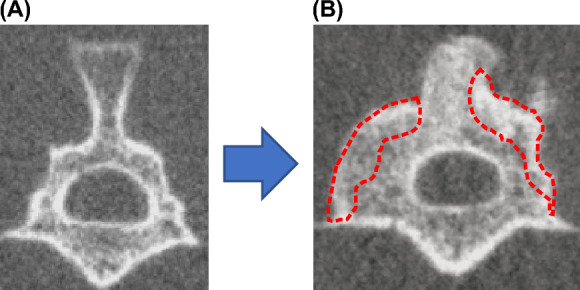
(A) Measurement of the volume of bone formation using Image J. (B) Determination of bone fusion by CT imaging.
